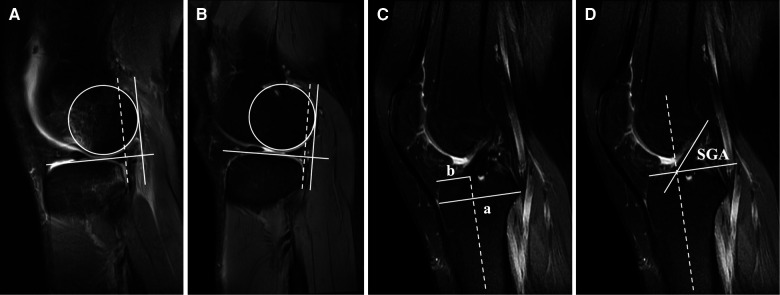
For MRI measurement (A) preoperative and (B) postoperative LATS, the slice showing the most medial edge of the fibula was chosen. Following that, a best-fit circle tangent to the lateral posterior femoral condyle border was first drawn. Along the posterior margin of this circle, a straight line was depicted vertical to the tibial plateau, and another parallel line (dotted line) was drawn across the posterior cortex of the tibia. The distance between these two lines was defined as the amount of LATS. (C) AP ratio was defined as the distance between the anterior tibial margin to the center of tibial graft insertion/the tibia's total AP distance (b/a). A line vertical to the tibia's axis (dotted line) was drawn from the anterior tibial cortex to the posteriormost part of posterior intercondylar margin to confirm the tibia's total AP distance. (D) SGA was defined as the angle between the tangent to the graft and a line perpendicular to the tibia's long axis (dotted line). LATS, lateral anterior tibial subluxation. AP ratio, anteroposterior ratio; SGA, sagittal graft angle.
